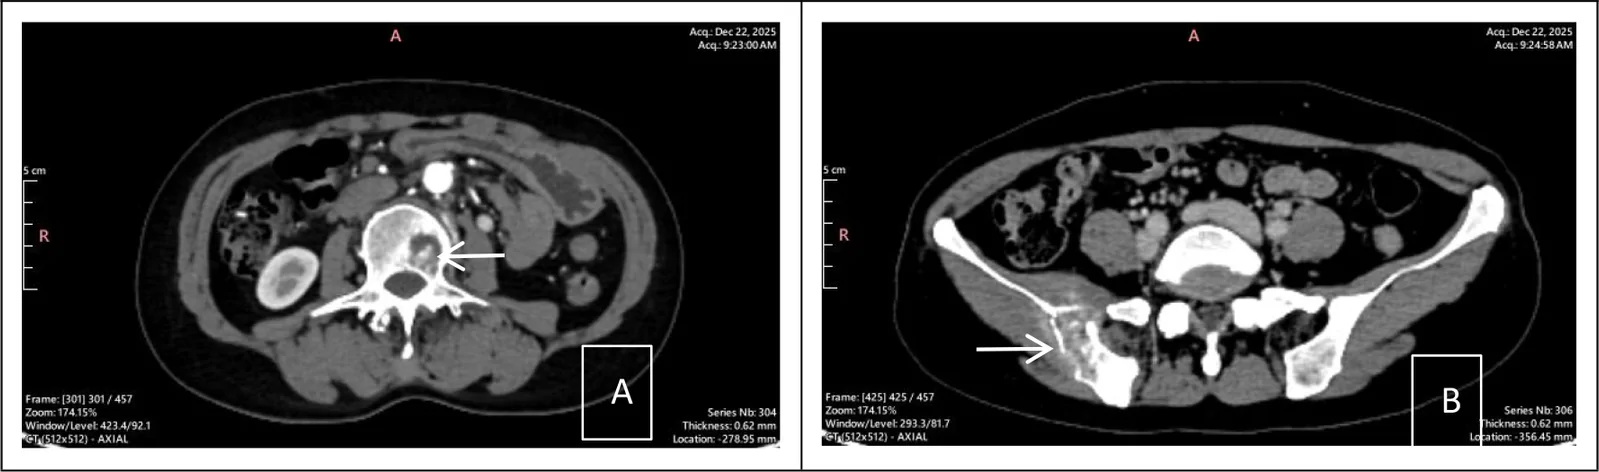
Contrast - enhanced CT imaging findings. (A) Heterogeneous enhancement in the bone destruction zone within the lumbar vertebral body (as indicated by arrows). (B) Heterogeneous enhancement in the bone destruction zone of the right ilium and the local soft tissue mass.
